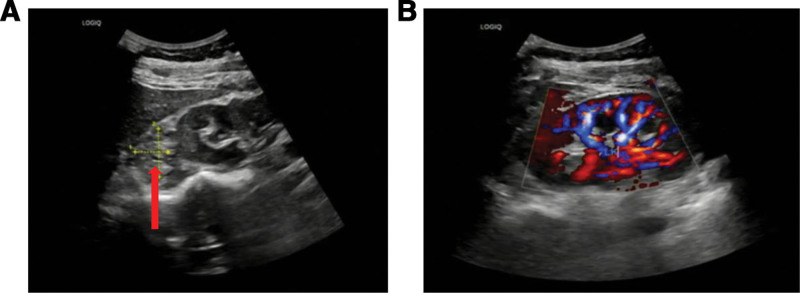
Equivocal ultrasound finding at 4-year follow-up.
